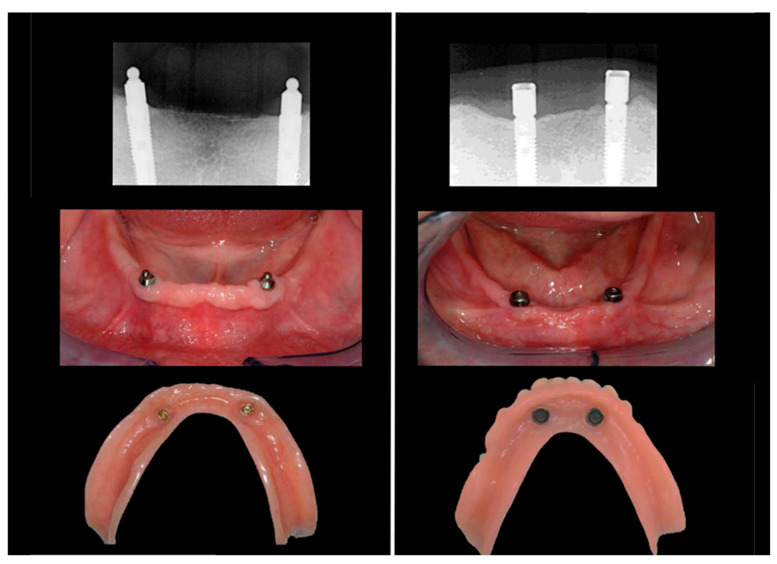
Radiographical and clinical example an overdenture retained on 2 non-connected implants on either 2 ball abutments (left) or 2 locator abutments (right).
